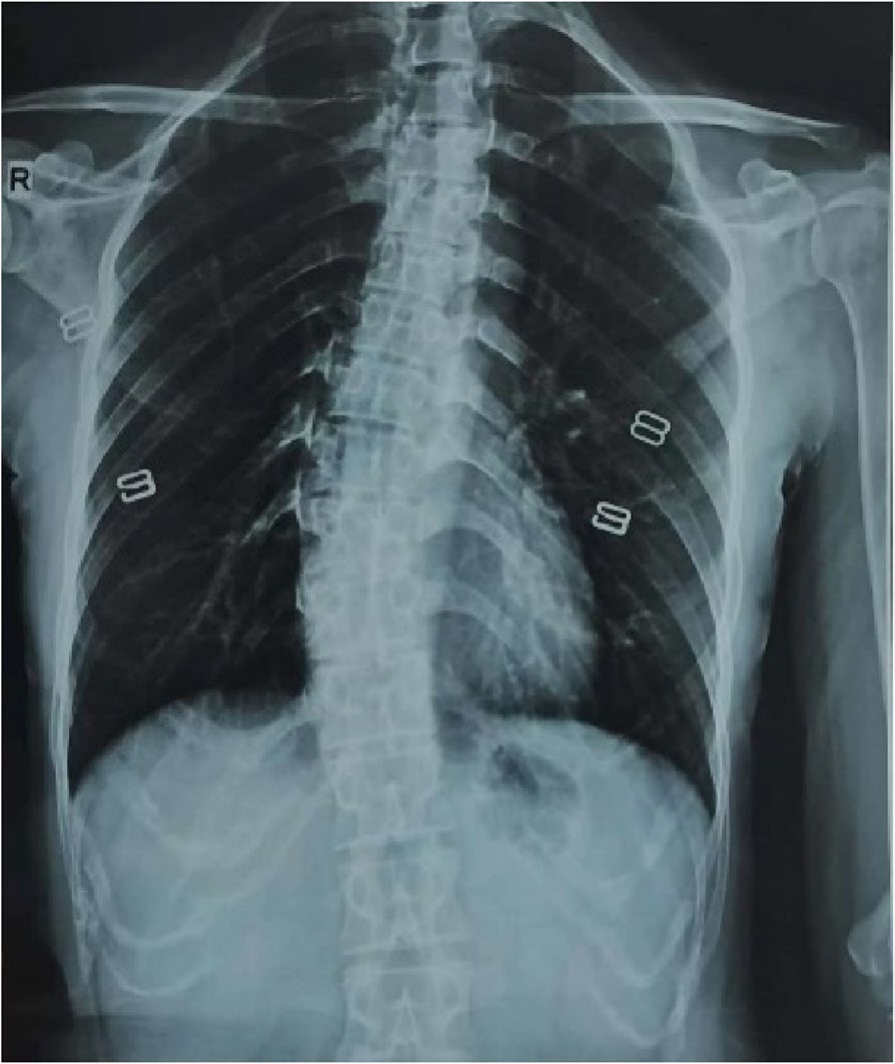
Spine X-Ray: scoliosis of approximately 20°
